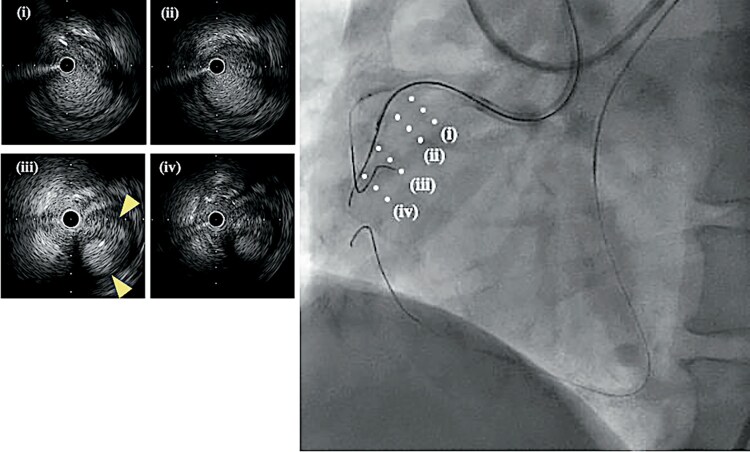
Intravascular ultrasound imaging. *(A–D)* Intravascular ultrasound images taken at each step. *(A*, *B)* Advancement of the guidewire into the true lumen. *(C*, *D)* Advancement of the guidewire into the extravascular space. The arrowheads indicate the typical appearance of a lotus root.
